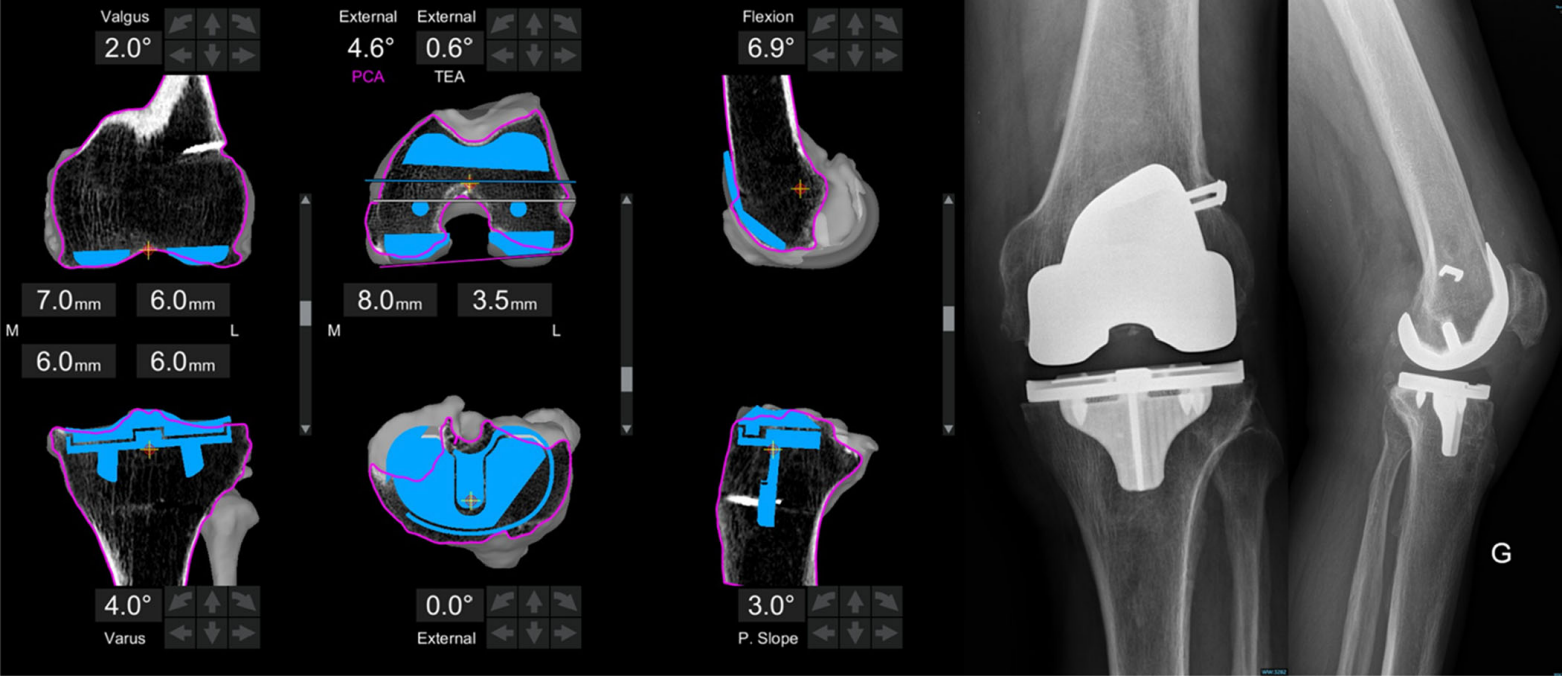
Screenshot from the robotic system of a planning for functional knee positioning following the principles of functional alignment and the related postoperative radiographic evaluation. PCA, posterior condylar axis; TEA, transepicondylar axis.
